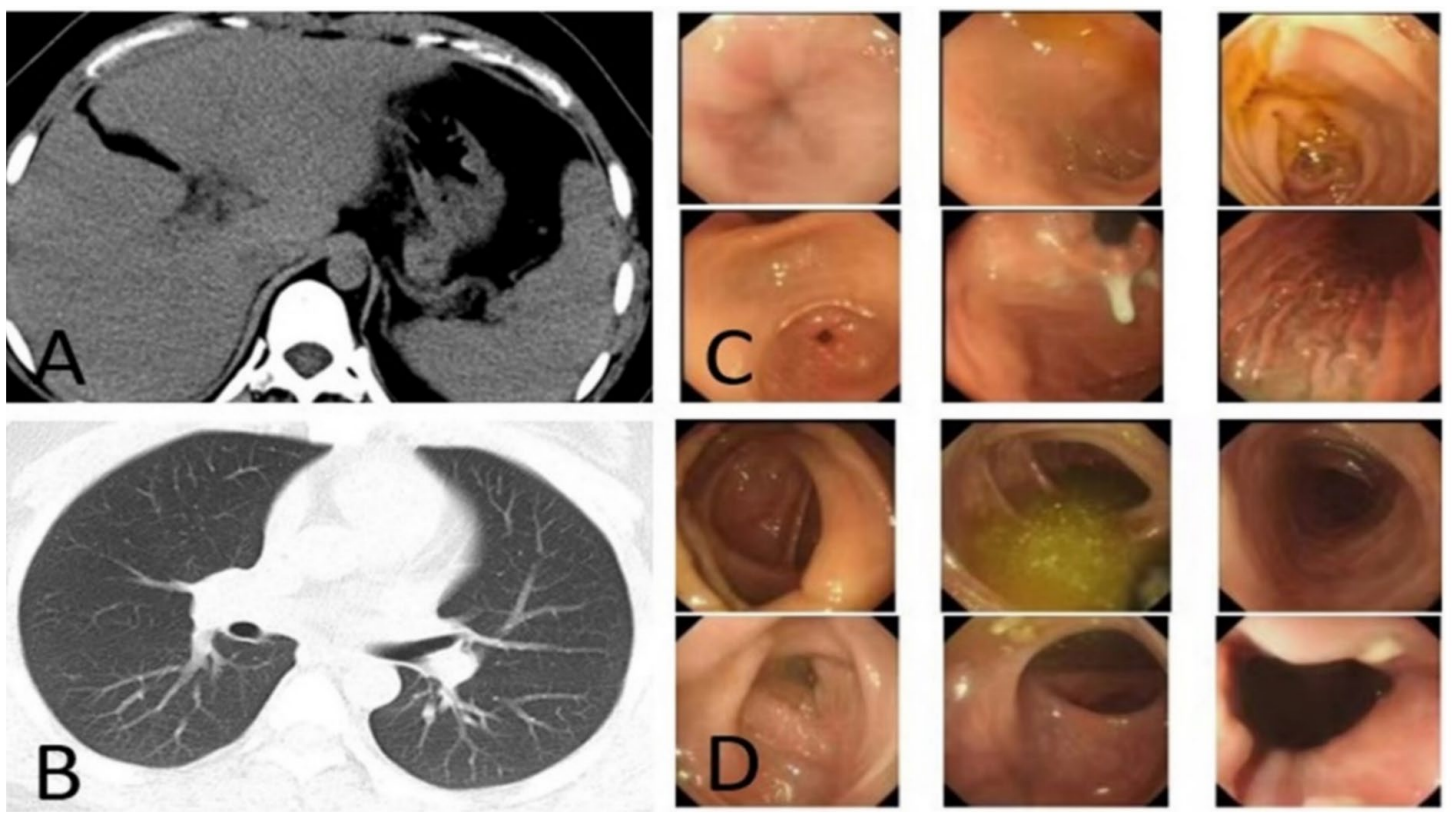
(A) CT image after resection the S4 segment of PHNET, (B–D) first post-operative negative images of chest CT, gastrointestinal endoscopy, and colonoscopy.
